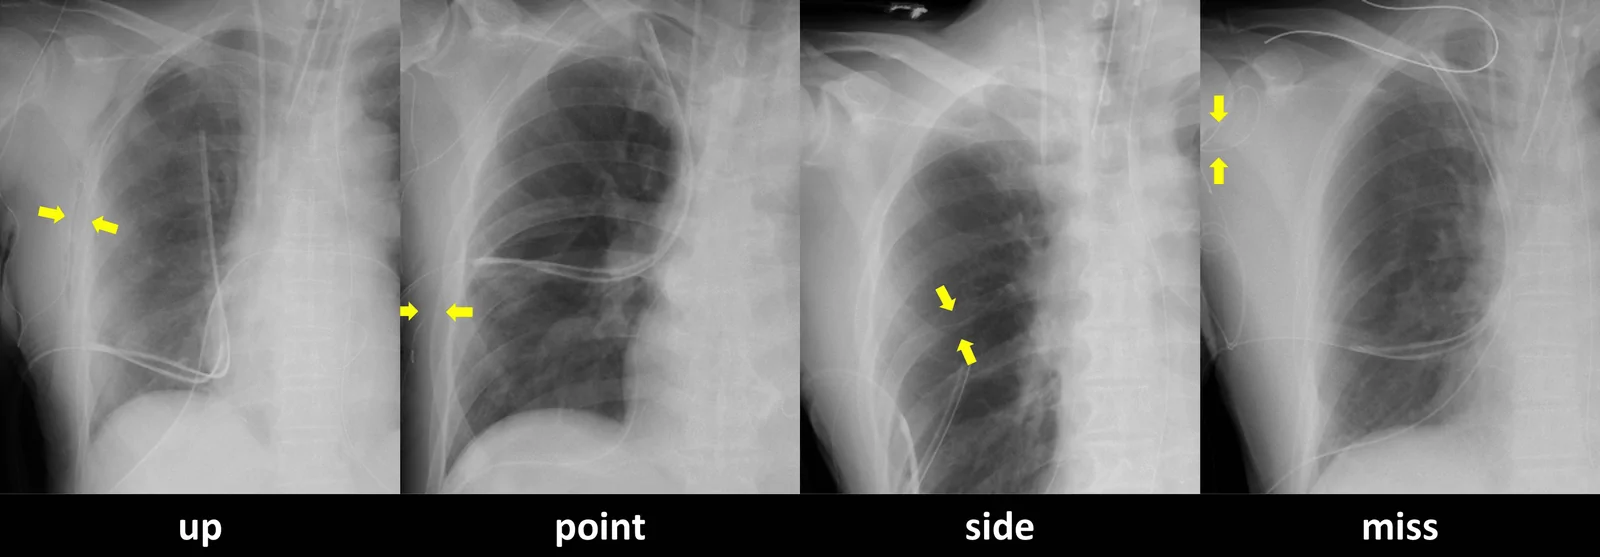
Representative postoperative chest radiographs of each catheter alignment category The catheter is indicated by the two yellow arrows. Catheter alignment was assessed using the outer contour of the rib as the anatomical landmark. The representative "up" image shows the catheter following the outer contour of the rib. In the "point" image, the catheter tip is projected at the outer edge of the rib. In the "side" image, the catheter tip is projected ventrally or dorsally relative to the rib. In the "miss" image, the catheter tip is projected within the soft tissue away from the rib.
